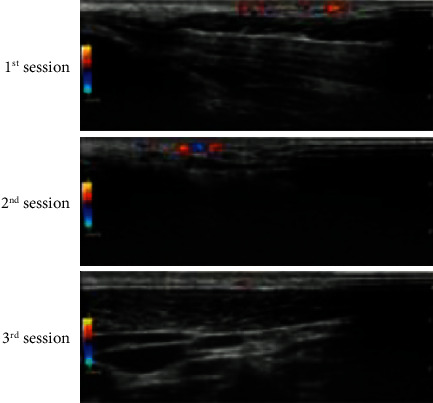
Changes in skin thickness and density before and after treatment with PWS in the high-frequency ultrasound skin mode. Before treatment, compared with the normal skin on the opposite side, the dilated deformed capillaries in PWS patients increased significantly, from the superficial layer of the dermis to the deep layer. After three HMME-PDT treatments, the deformed vessels in the superficial dermis of the skin lesions were significantly reduced, and a small number of star-shaped blood flow signals were seen.
